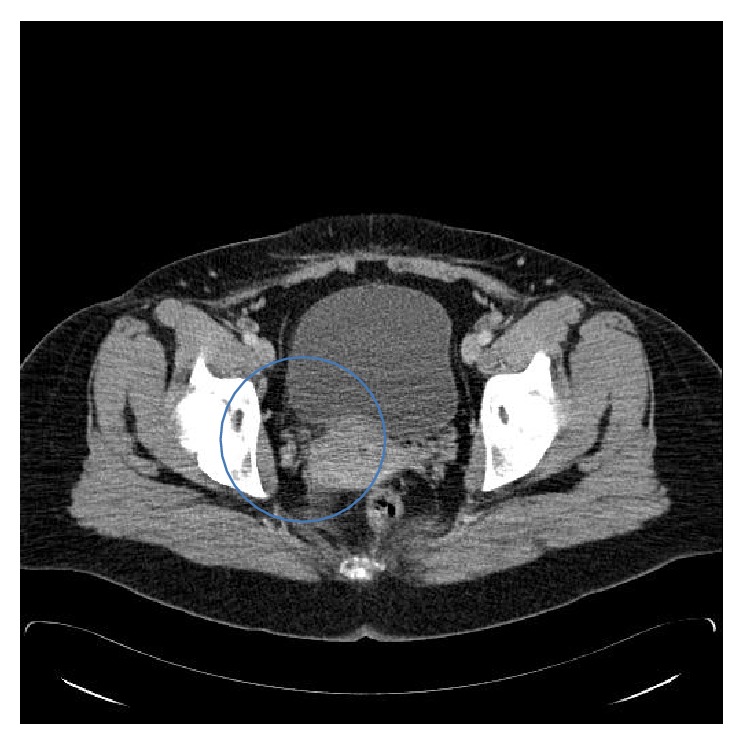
CT at 6 months postpartum. The previously visible right-sided pelvic mass is no longer present.
